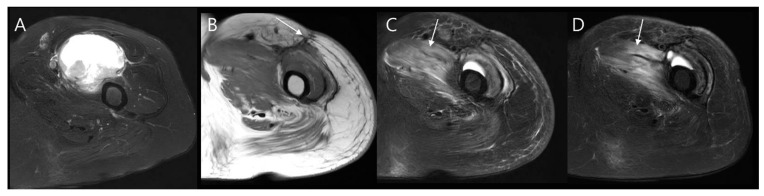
Denervation-related muscle changes. (A) Preoperative axial T2-weighted fat-suppressed MR image shows a synovial sarcoma in the proximal thigh. A wide excision with femoral vessel ligation was performed. (B) Postoperative axial T1-weighted image taken 7 months later reveals a fibrotic scar at the incisional site (arrow). (C) Axial T2-weighted, fat-suppressed image shows edematous change due to denervation in areas innervated by the femoral nerve (arrow). (D) Axial T2-weighted, fat-suppressed image taken 1 year postoperatively shows partial regression of the edema, along with volume loss in the affected muscle (arrow).
